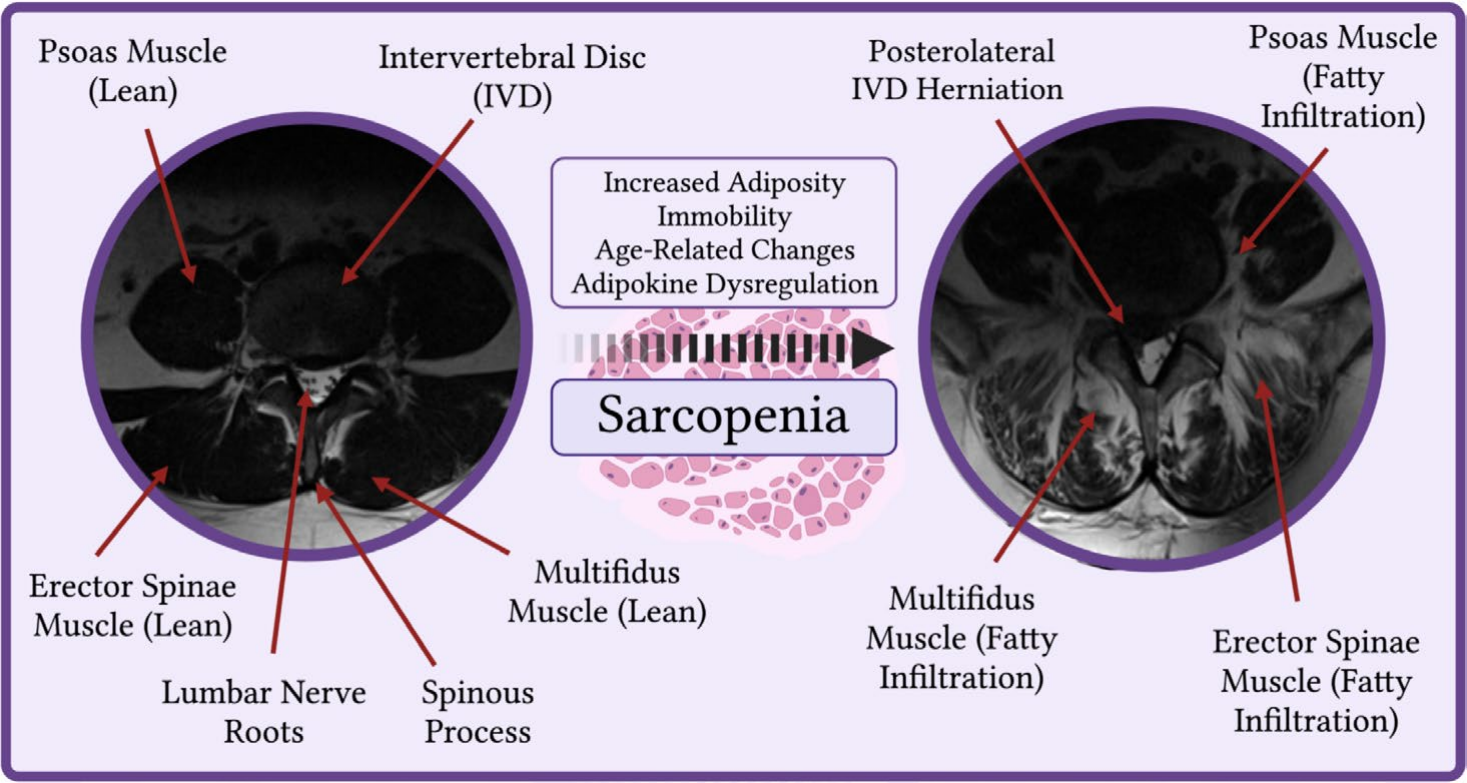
Axial T2‐weighted slice of L3‐4 spinal level on magnetic resonance imaging (MRI) highlighting comparative lean and sarcopenic paraspinal muscles (psoas, multifidus, erector spinae) in two patients, in addition to a right‐sided posterolateral herniation with compression of adjacent lumbar nerve roots.
